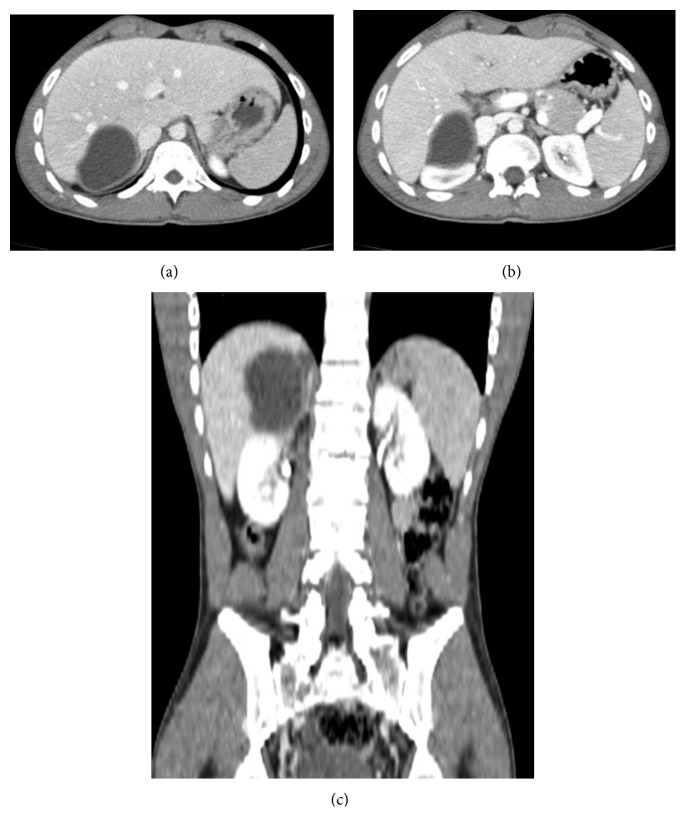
Axial (a, b) and coronal (c) contrast-enhanced CT images. A lesion with lobulated contours and a size of 70 × 70 mm that was compatible with a hydatid cyst. The lesion originates from the hepatic segment V-VI and gives off exophytic extensions in the posterior-inferior direction.
